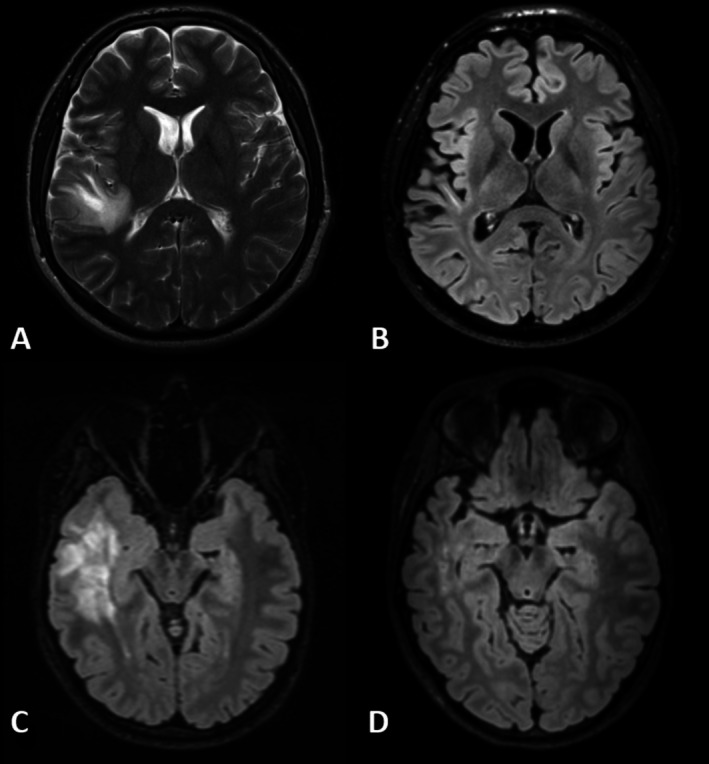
(A) Patient 1 (male, 20 years‐old) with MOGAD and ADEM manifestation in right temporal and parietal lobe (6 years before baseline MRI). (B) Follow‐up MRI scan of patient 1 in the context of our study (6 years later) demonstrating marked reduction of the tumefactive lesion. (C) Patient 2 (female, 19 years old) with MOGAD and ADEM manifestation in right temporal lobe. (D) Follow‐up MRI scan of patient 2 within our study (6 months later) showing almost complete regression of the lesion.
